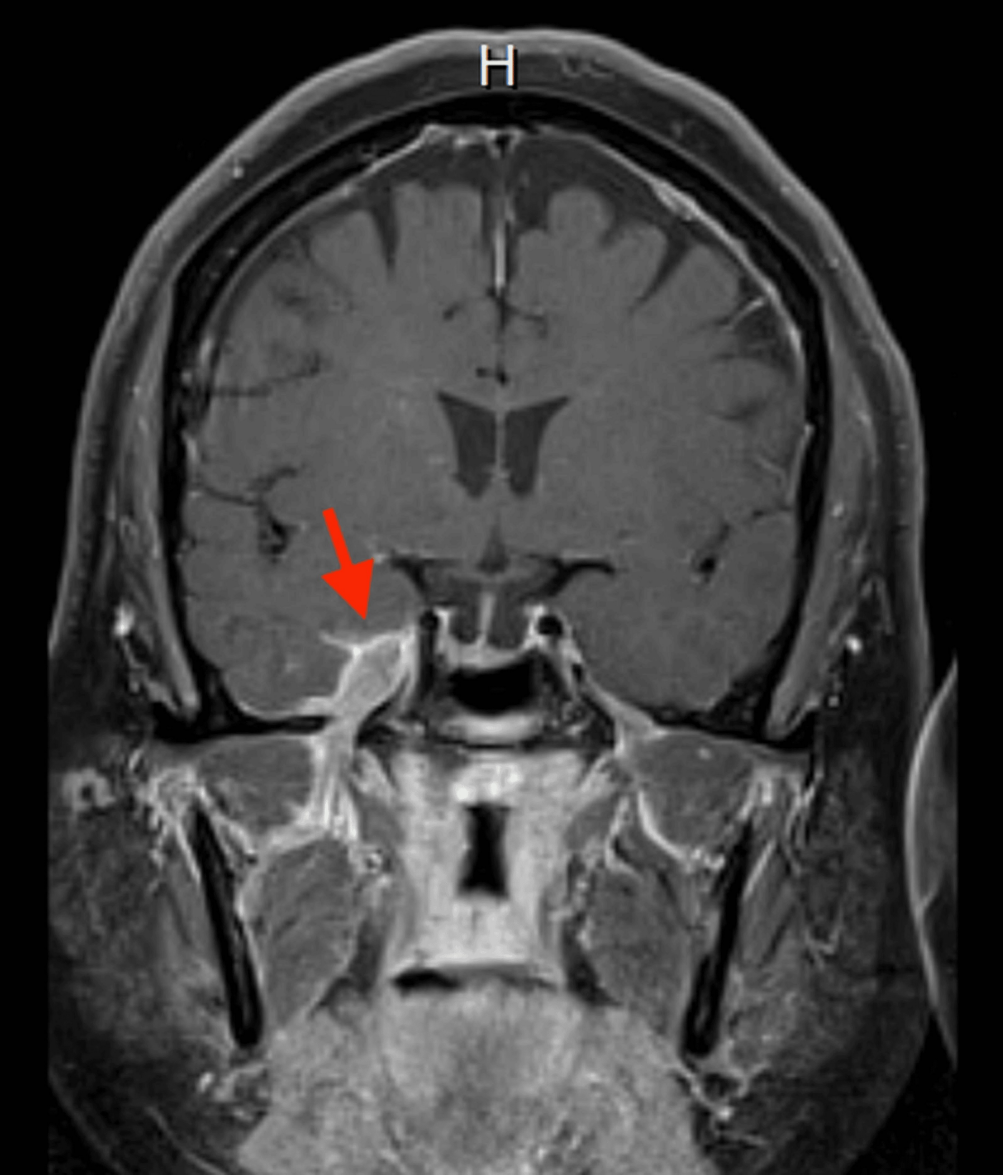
Contrast-enhanced MRI revealing enhancing lesion in the right parasellar region involving Meckel's cave.
